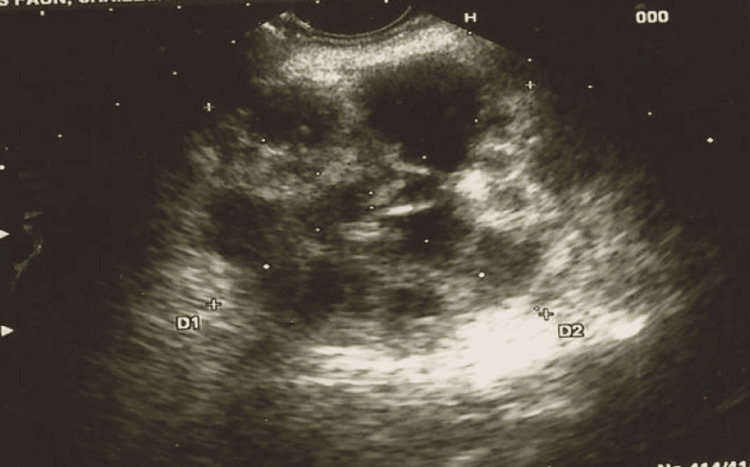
Endosonography Endosonography demonstrated the presence of a cystic mass (marked by the + sign), in the body of the pancreas, providing crucial information for further investigation.
